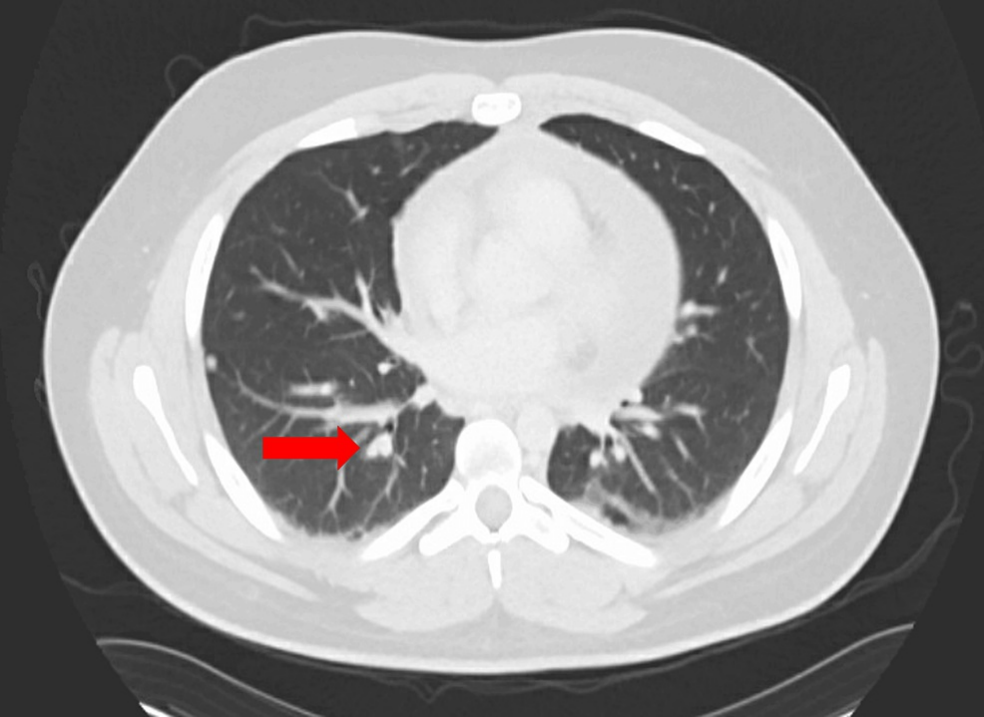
CT scan showing a small right pulmonary nodule (red arrow).
